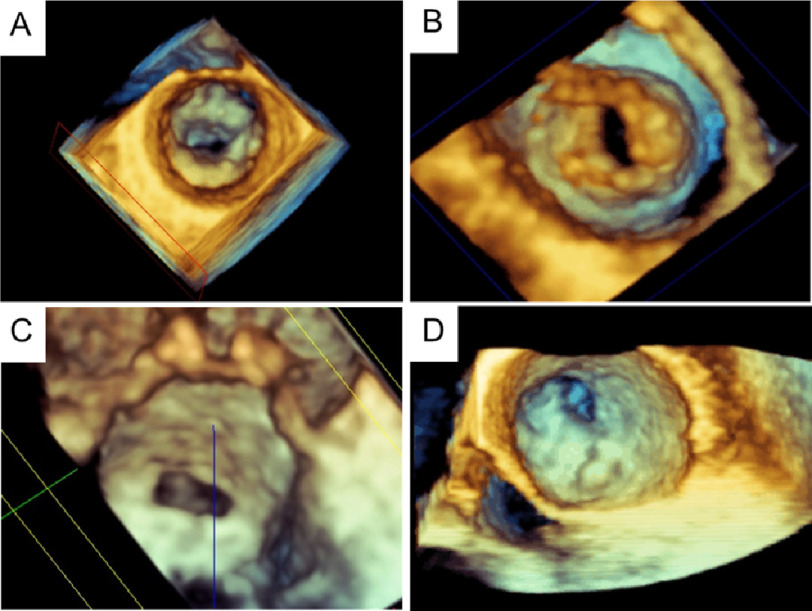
Three-dimensional transesophageal echocardiography images of rheumatic mitral stenosis. Mitral valve seen from the left atrium (A). En face view of the mitral valve from the left ventricle (B). Rheumatic mitral stenosis with symmetric commissural thickening. Severe rheumatic mitral stenosis with significant thickening of anterolateral commissure, leading to an eccentric valve orifice (D).
